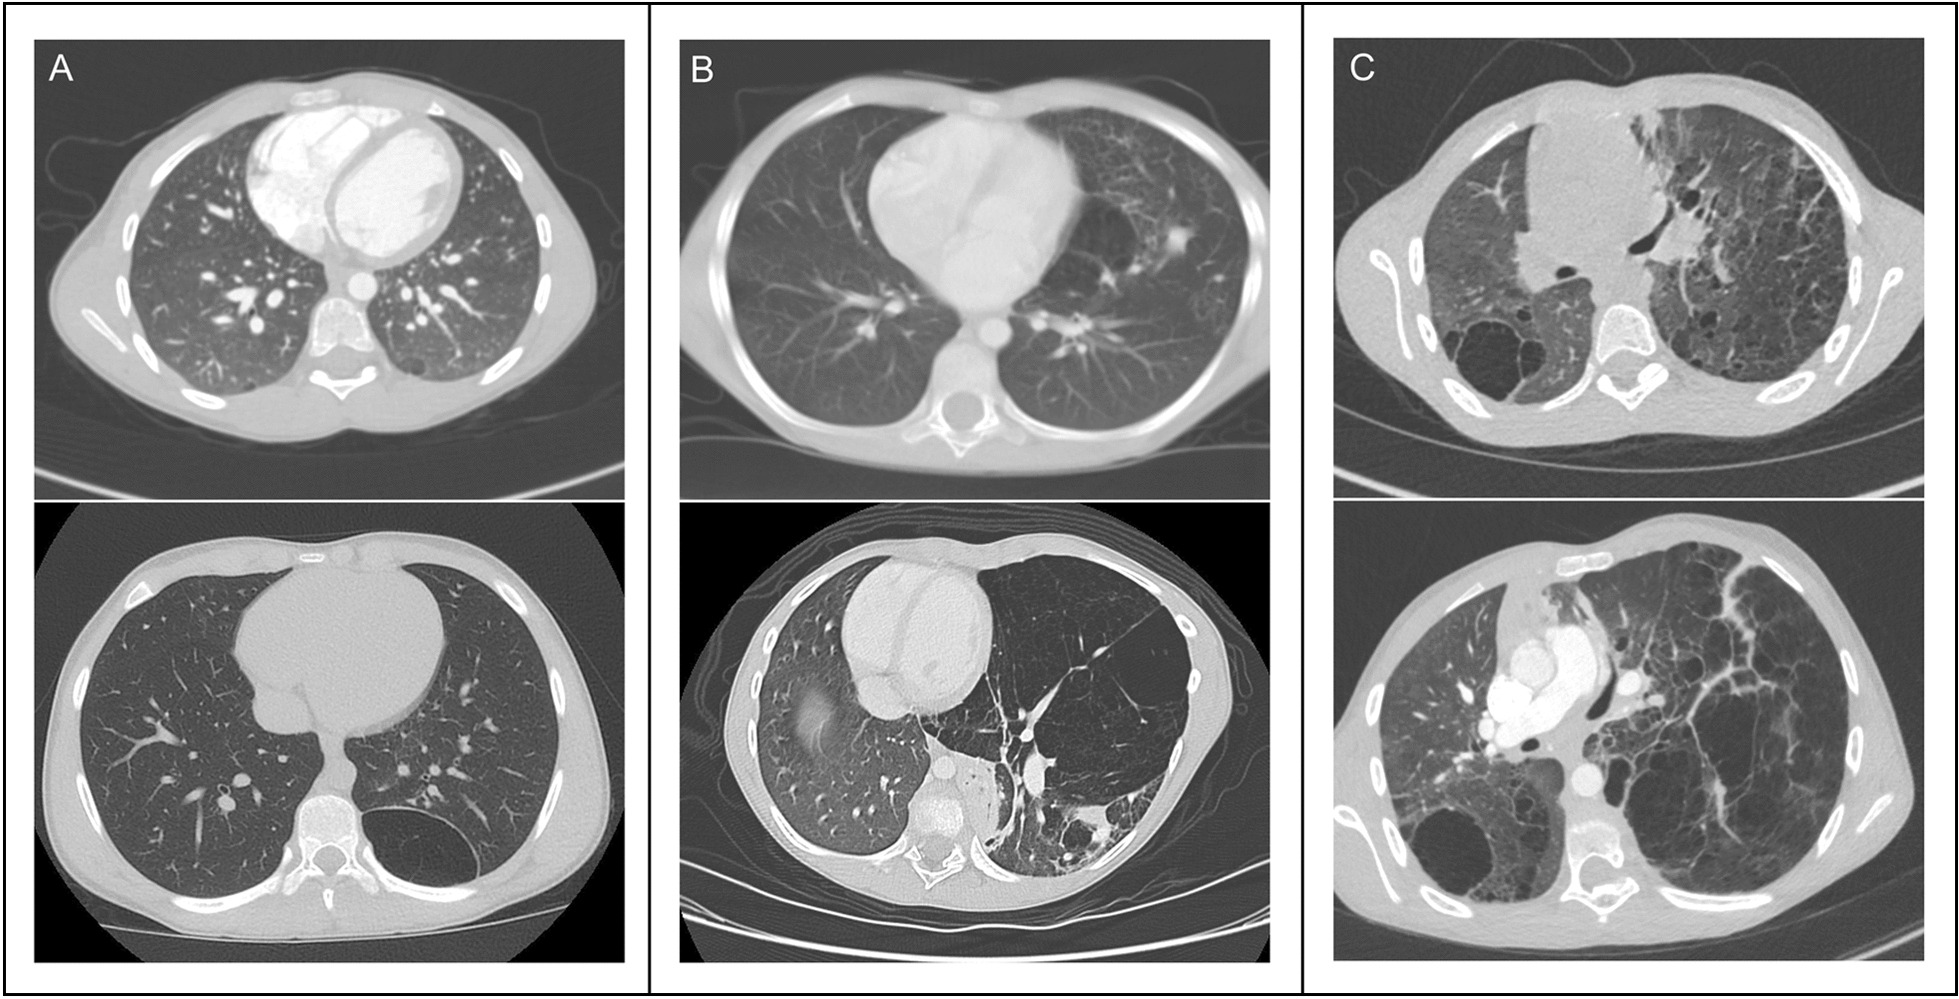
Radiographic progression of lung disease in three individuals A, B, C at seven years old (top) and after three or four years (bottom)
